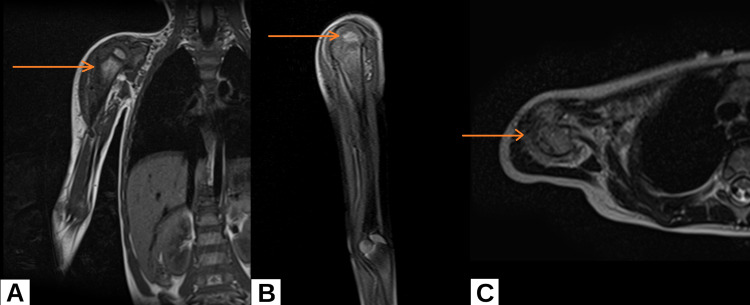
MRI of the patient coronal view (A), sagittal view (B), and transverse view (C). MRI shows an ill-defined, infiltrative, aggressive-looking, hyperintense, enhancing mass arising from the proximal metadiaphysis region of the right humerus with permeative destruction of the cortex and associated periosteal reaction/enhancing soft tissue component. MRI: magnetic resonance imaging
